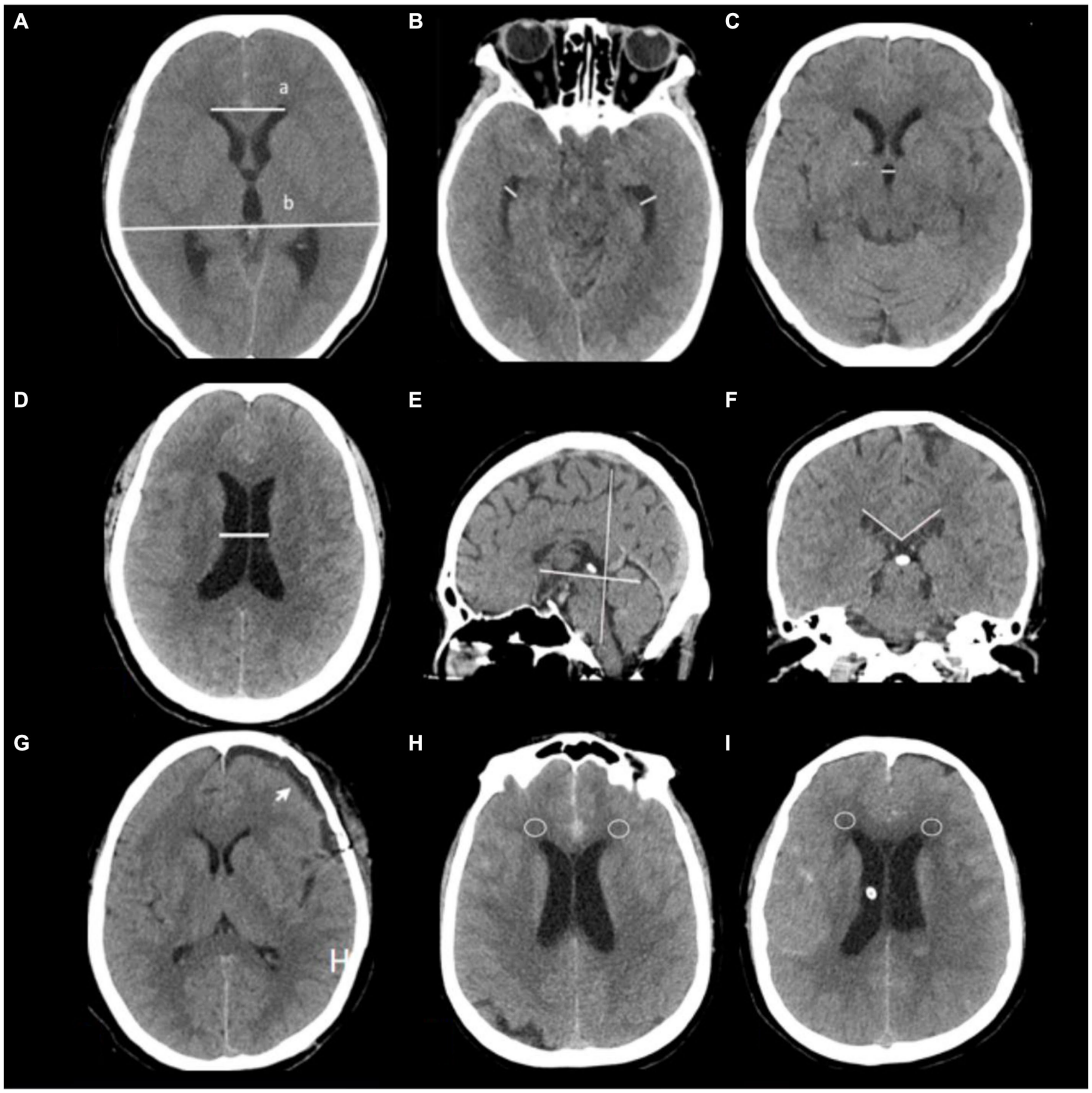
Radiological features are depicted in this study. (A) Evaluation of hydrocephalus entailed the use of CT scans and Evans’ index, which measures the ratio between the maximum width of frontal horns (a) and the maximal internal diameter of the skull (b) at Monro’s foramens. (B) Temporal horn widths are measured strictly at the ventricular prominence level. (C) Third ventricle width, (D) Ventricular width refers to the narrowest distance between walls on both sides of the lateral ventricle body, measured axially; CT slices should be taken where the cella media first appears unobscured by other regions (thalamus) (G) subdural hygroma after surgery (arrow). (E,F) Corpus callosal angles are measured on coronal planes perpendicular to each subject’s anteroposterior commissure plane on their posterior commissure. (H,I) CT values around anterior horns intervals a patient 7 days after admission (circles place).
